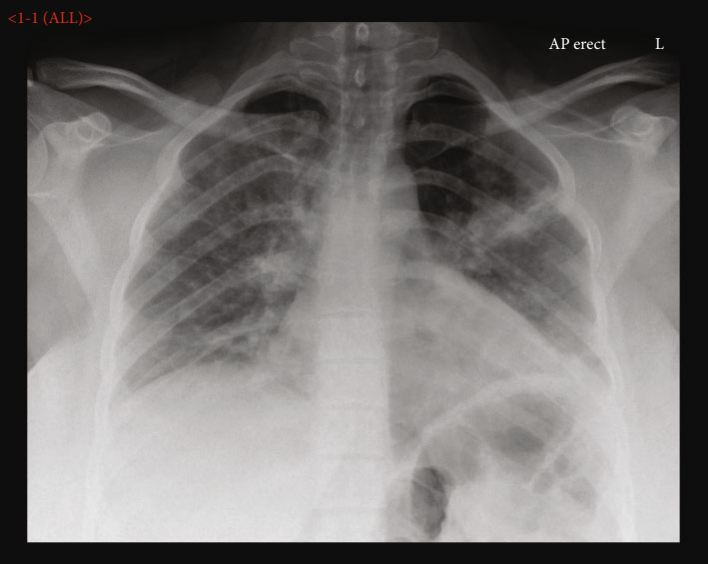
Chest X-ray on admission to the hospital prior to intubation.
